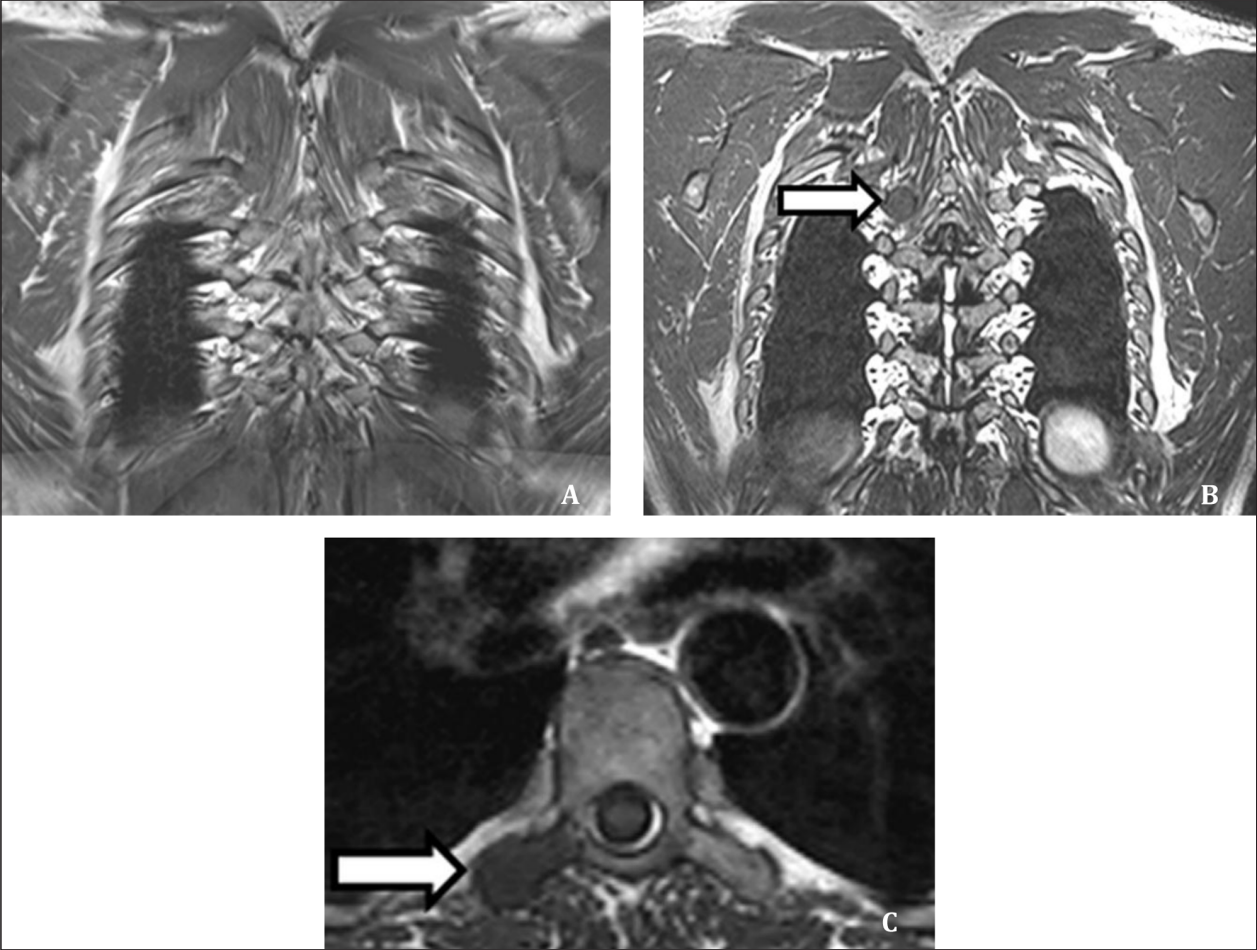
Detection of a BM in a 63 year old PCa patient. The lesion was missed by both readers on 2DT1 sequence (A) but correctly identified by both on 3D T1 sequence (B). Axial reconstruction obtained with multiplanar reformation at same level confirms the presence of a BM (C). Reprinted from ‘Whole-body 3D T1-weighted MR imaging in patients with prostate cancer: feasibility and evaluation in screening for metastatic disease’ Pasoglou V., Michoux N., Peeters F., Larbi A., Tombal B. Omoumi P., Vande Berg B., Lecouvet F.E, *Radiology*. 2015 Apr; 275(1): 155–66. [43] With the permission of RSNA.
